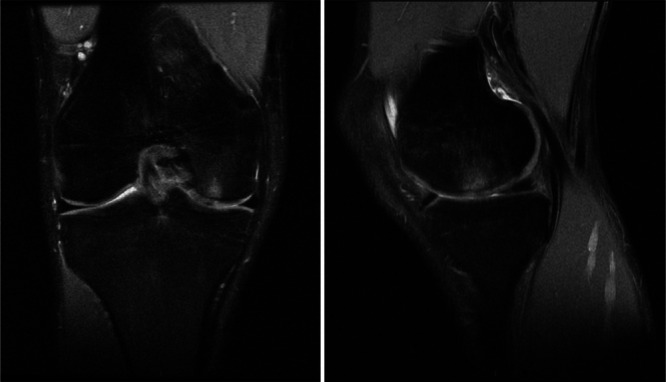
Subchondral lesions are a common finding after cartilage procedure. In this example, magnetic resonance imaging (MRI) of perifocal bone marrow oedema‐like signals are detected 5 years after the matrix‐associated autologous chondrocyte transplantation (MACT) procedure for the chondral lesion of medial femoral condyle in a 20‐year‐old male patient, but the patient presented a full symptomatic and functional recovery at the time of MRI.
